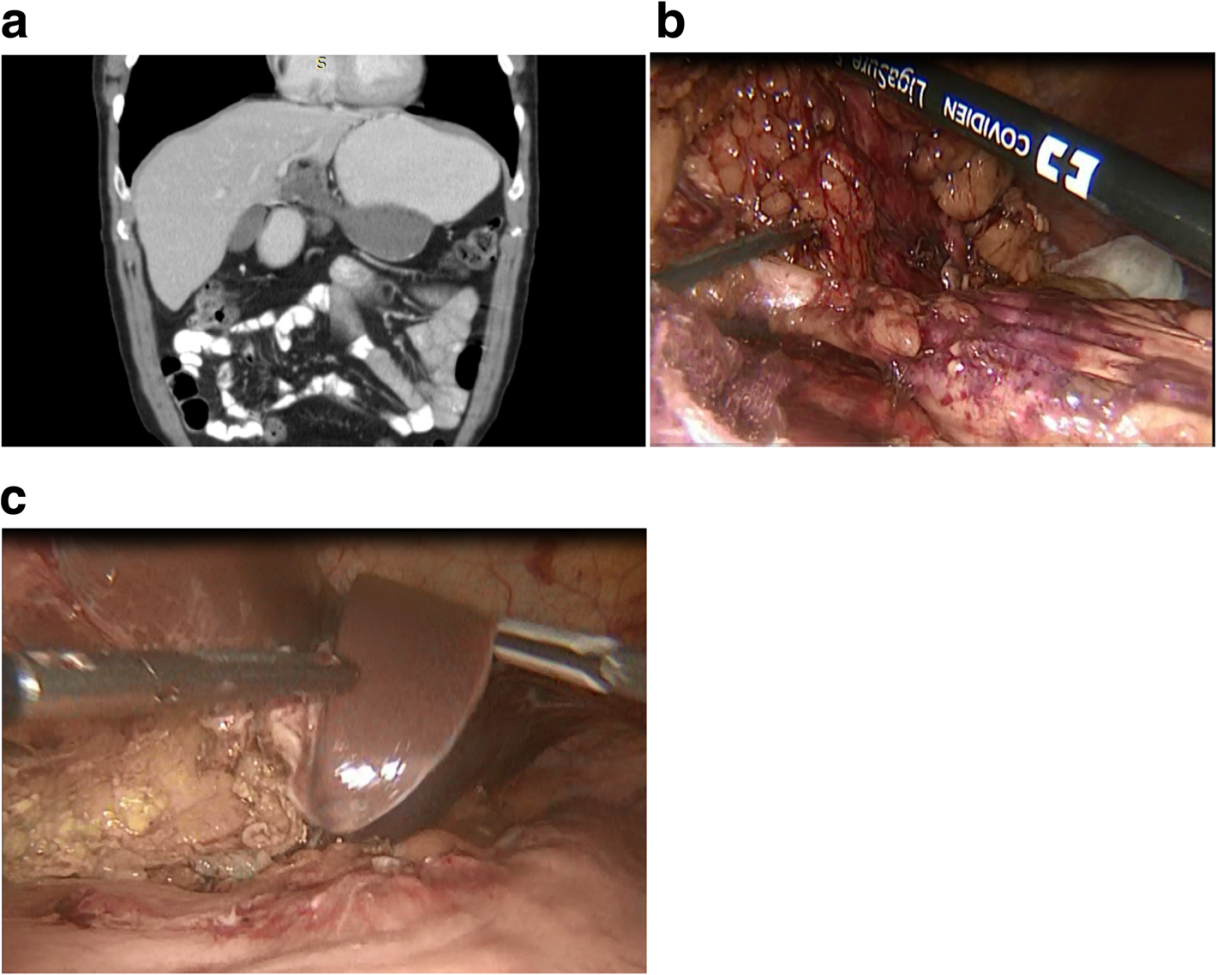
a CT imaging of type 3 cystic hydatid cyst with exophytic distension from the left lobe of the liver, compressing the stomach posteroinferiorly and operative figures of partial pericystectomy in this case (b); the appearance at the end of the operation (c)
